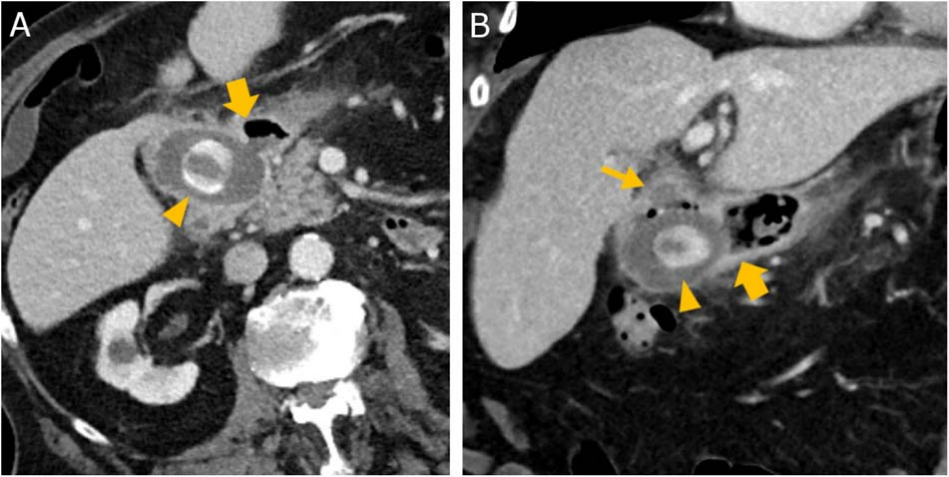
Abdominal CT in axial (A) and oblique paracoronal reconstruction (B); gallstone in the duodenal bulb (arrowhead
